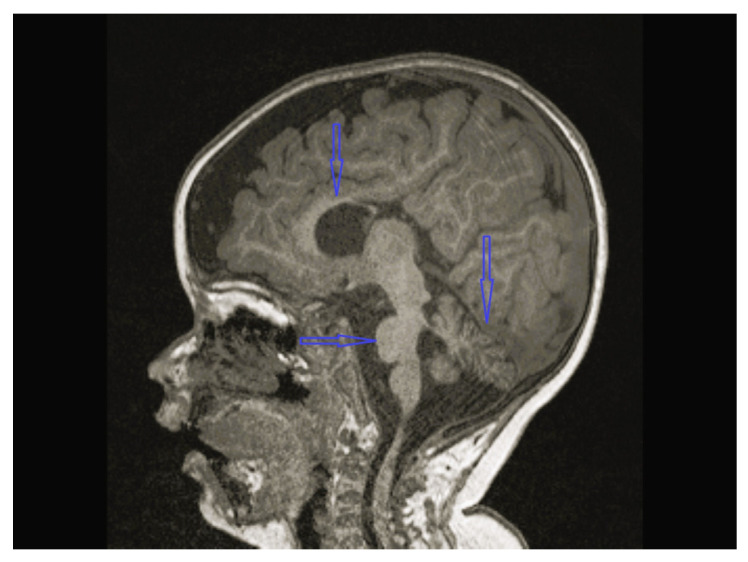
Brain MRI of case 8 revealing vermian, corpus callosum and brainstem hypoplasia.
